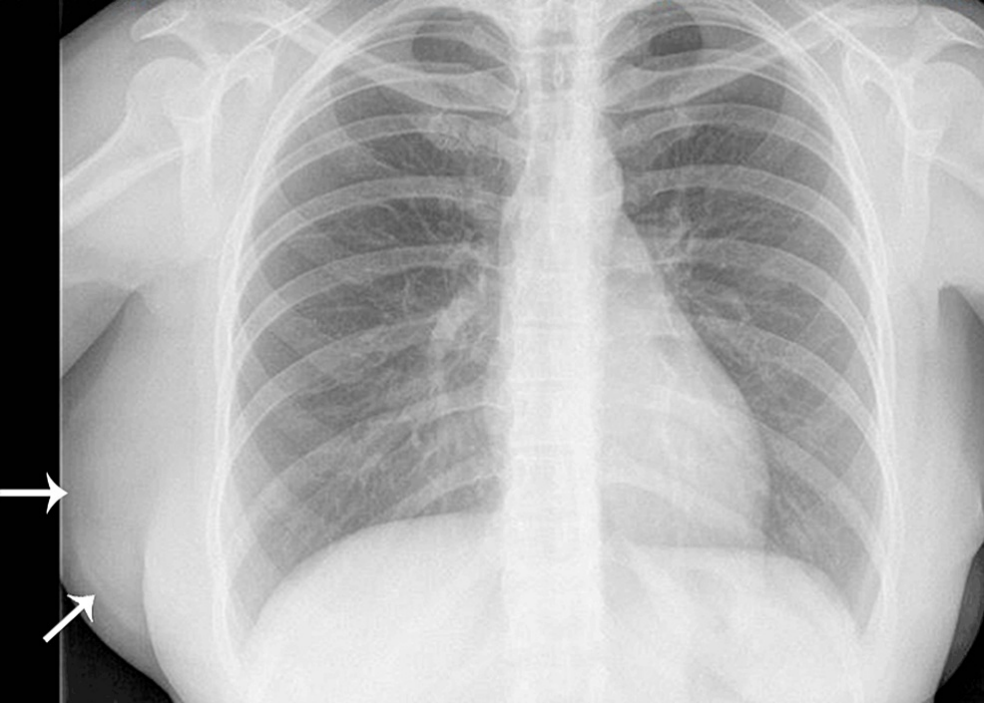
Chest roentgenography presented a clearly defined radiolucent homogenous mass in the the right lateral chest wall, with the same density as surrounding adipose tissue (arrows).
